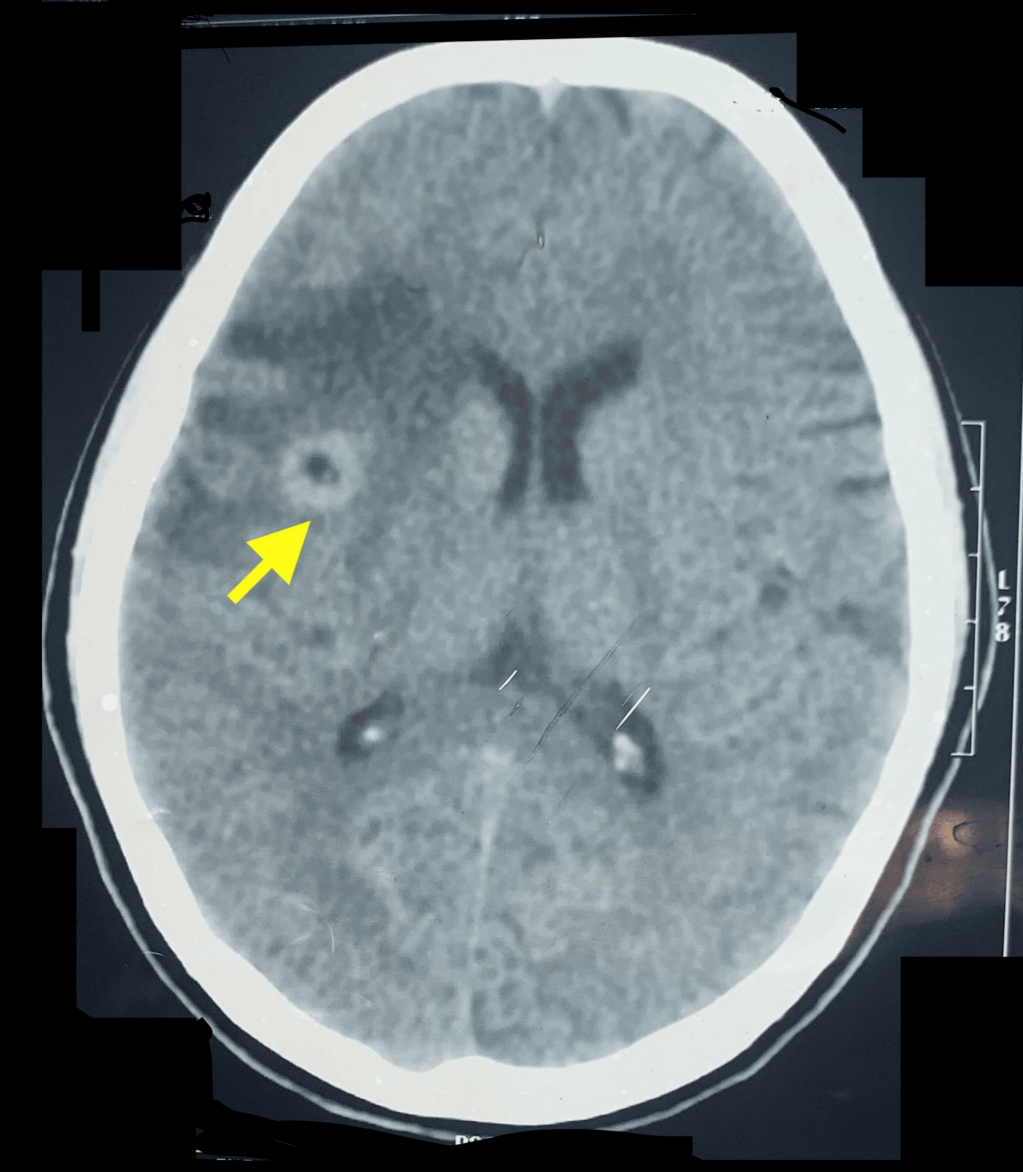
An unenhanced CT scan shows a single parenchymal cyst in the right frontal lobe of the cerebral cortex. Inside the cyst, there was a hyperdense dot with a ' hole-with-dot' sign; extensive edema around the cyst was also noted.
